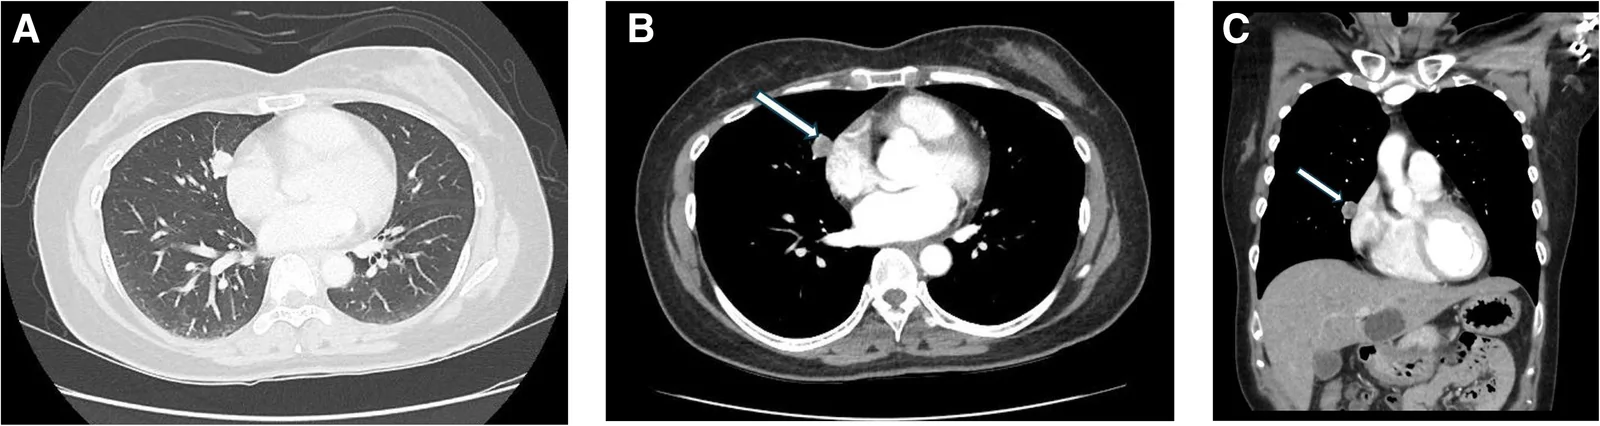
(A–C) Chest CT demonstrated a 1.6-cm, well-defined solid nodule (arrow) with inhomogeneous enhancement in the medial segment of the right middle lobe, immediately adjacent to the mediastinal pleura. Note the loss of the intervening fat plane between the lesion and adjacent pulmonary vasculature. CT = computed tomography.
